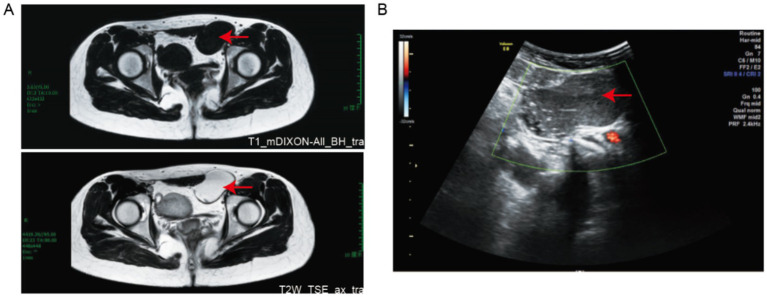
Preoperative MRI and transvaginal ultrasonography of the left inguinal lesion. (A) MRI demonstrating a well-circumscribed cystic lesion in the region of Hesselbach’s triangle, extending from the preperitoneal plane to the layer beneath the external oblique aponeurosis. The lesion measured approximately 6.2 × 3.4 × 5.5 cm and showed low signal intensity on T1-weighted imaging and high signal intensity on T2-weighted, fat-suppressed, and diffusion-weighted sequences, without definite contrast enhancement. No enlarged pelvic or inguinal lymph nodes were identified. (B) Transvaginal ultrasonography demonstrating a cystic lesion with internal linear and punctate hyperechoic structures and minimal blood flow signals along the cyst wall. Red arrows indicate the lesion.
